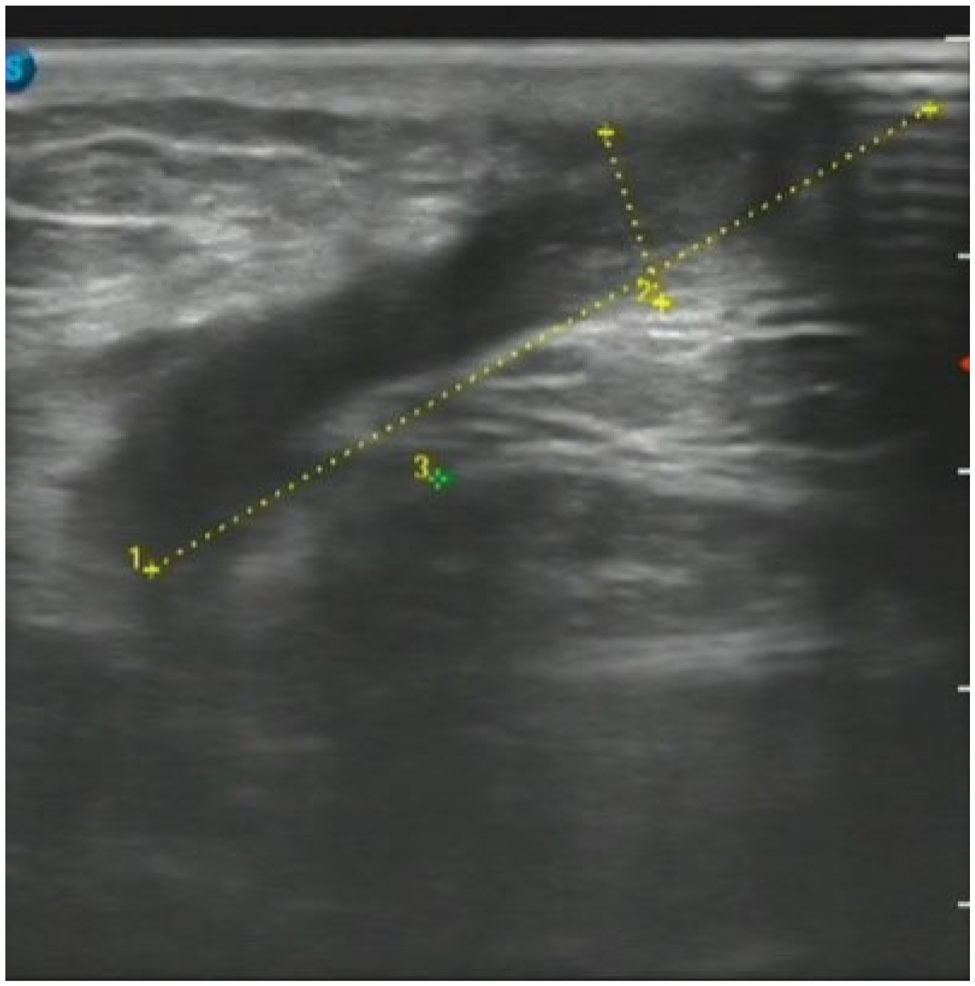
B-Mode Ultrasound Image of case 2 (b).
